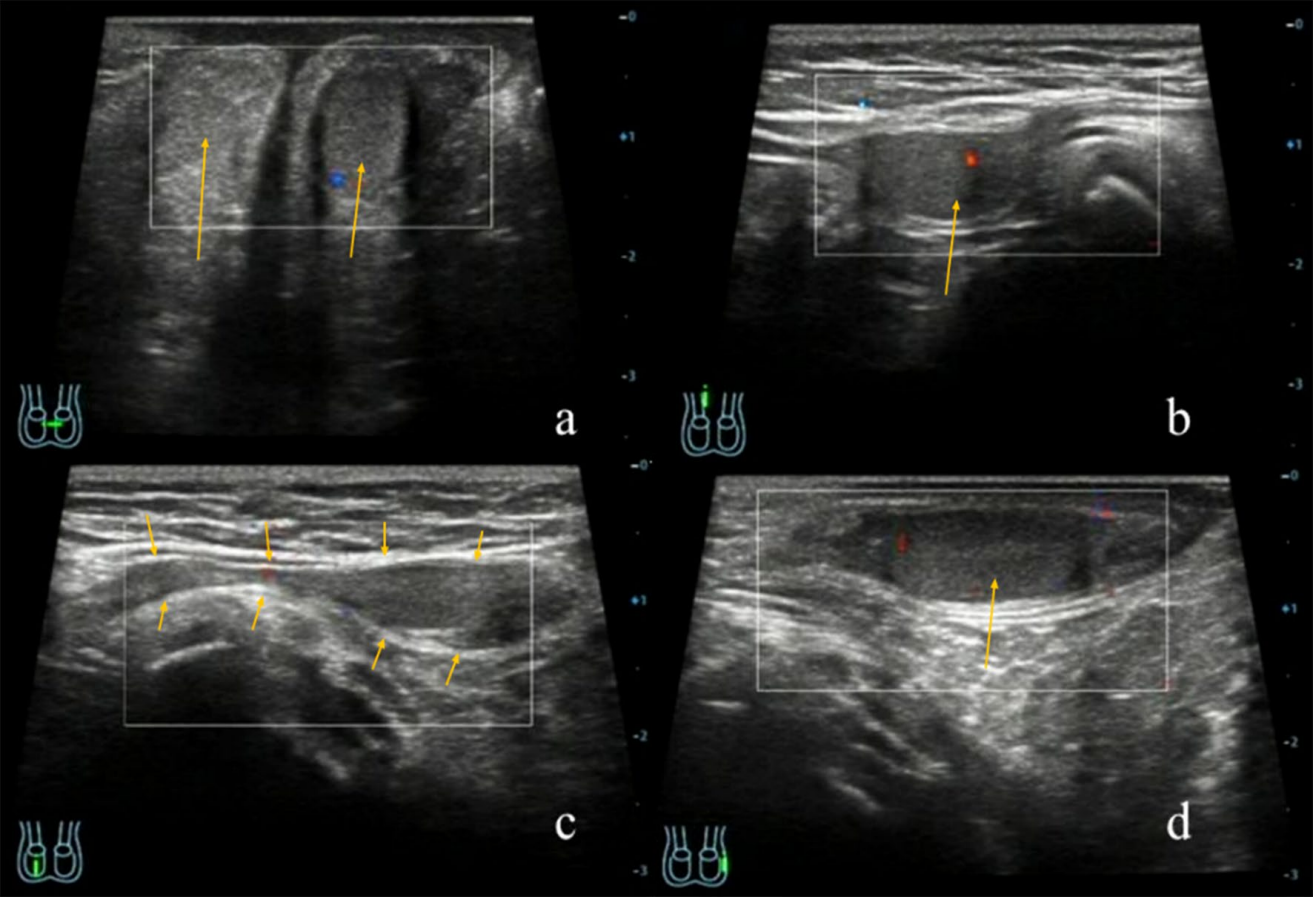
Ultrasound features of EAT on the right side of a 10-months-old boy. (a) The cross section of the scrotum has showed that the left testicular was in the left scrotum, and the right testicular echo was not in the right scrotum (b) and the upper part of the right inguinal canal near the inner ring showed the right testicular, and CDFI showed punctate blood flow signals inside (c) and the right testis can be moved down to the middle part of the right inguinal canal (d) and the CDFI of the left testis longitudinal section showed punctate blood flow signal in the left testis (arrows).
